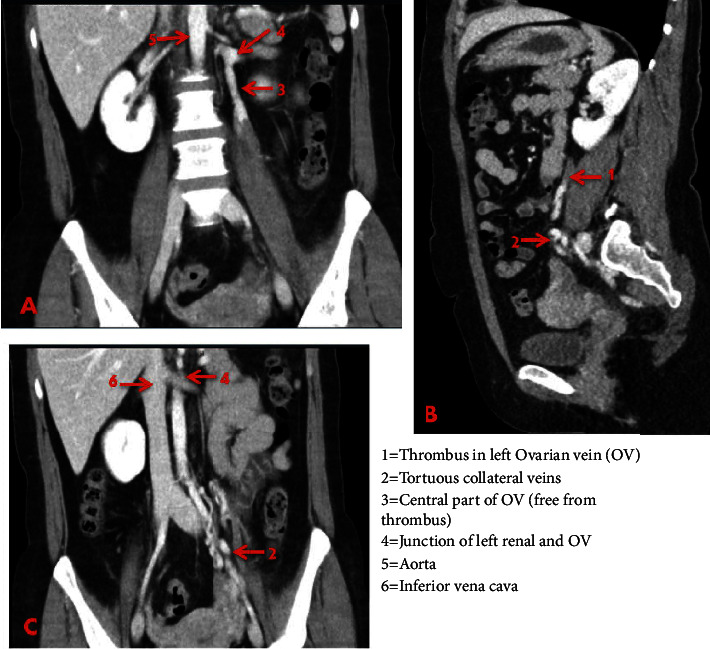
CT abdomen with contrast in venous phase showing thrombosis of the left ovarian vein and tortuous collateral veins. (A, C) coronal and (B) sagittal sections.
